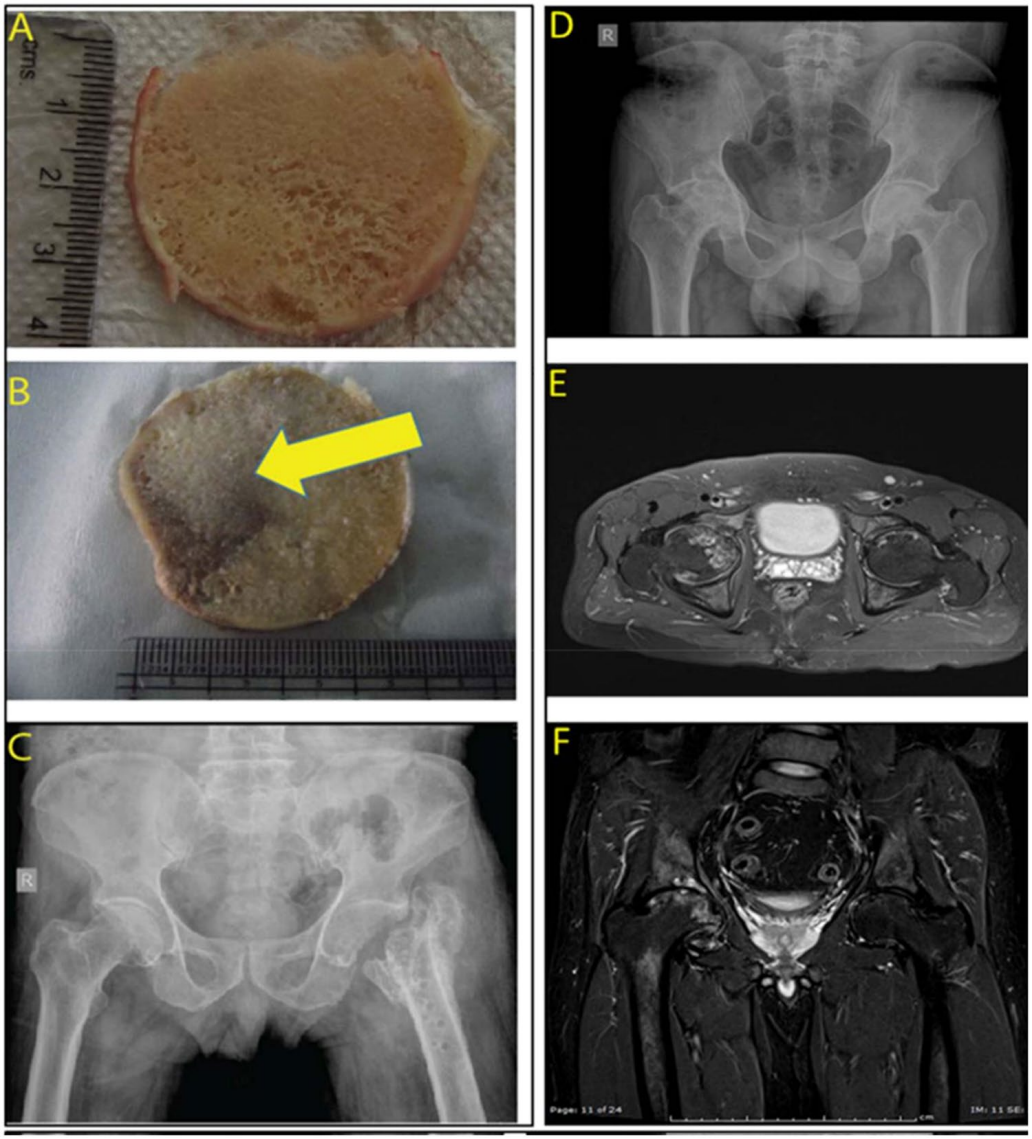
(A) Overview of representative AVNFH and control bone samples used in the study. (A) A representative gross image of a trabecular cross section of control bone. (B) Same as in A, but for AVNFH bone. Region affected by AVN is shown by the yellow arrow. (C) X ray image of the pelvic region from a control individual with fractured femur (D) X ray image of the pelvic region of a patient with Avascular Necrosis of the Femoral Head (AVNFH). (E,F) Magnetic Resonance Image (MRI of the pelvic region of a patient with AVNFH.
